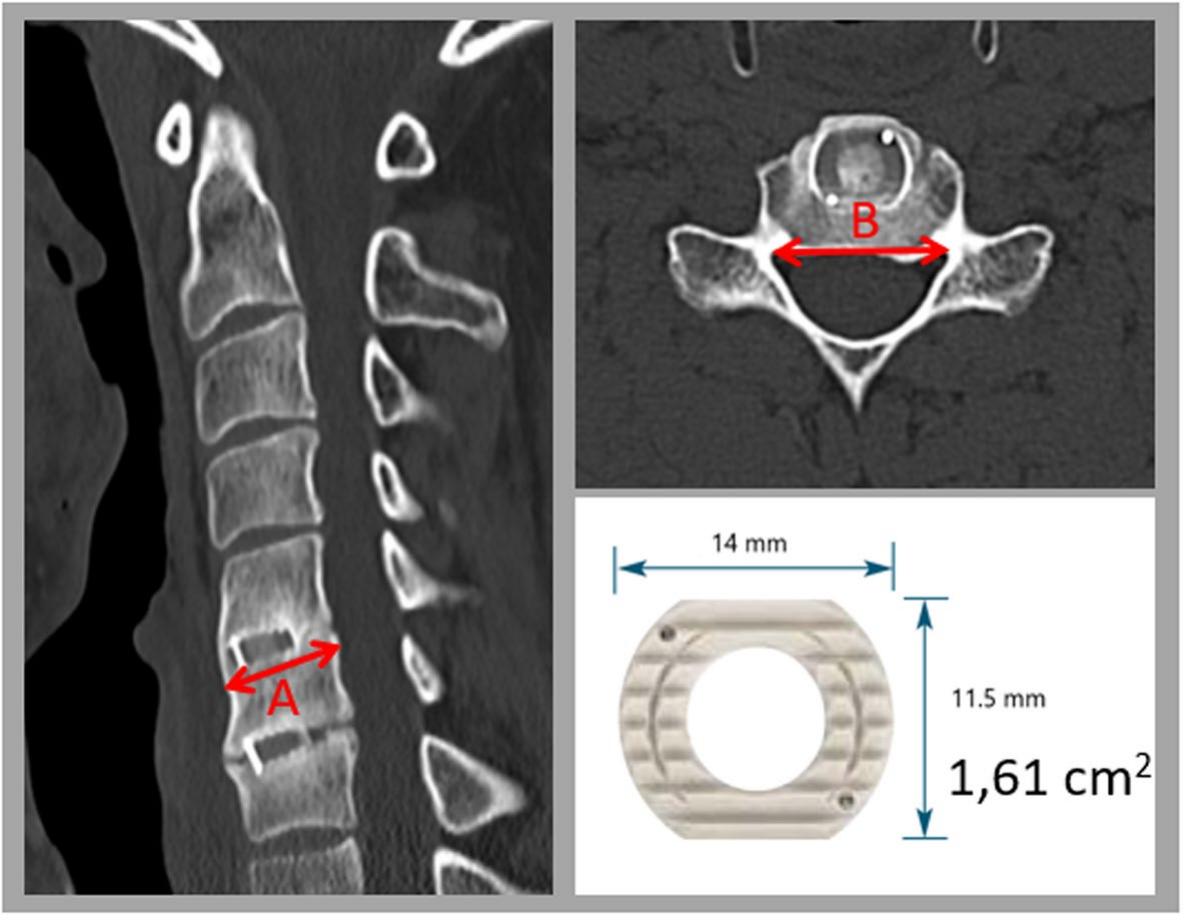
Procedure for measuring bone surface area of vertebral bodies immediately adjacent to implants on the basis of CT images obtained at 12 months post-surgery. A (cm) x B (cm) = surface area (cm^2^). All implants had the same surface area of 1.61 cm.^2^
